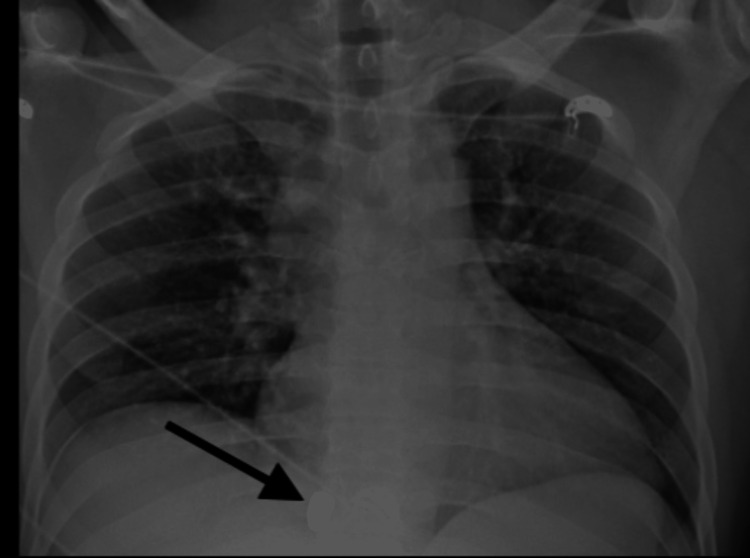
Supine AP chest radiograph demonstrates ballistic fragment projecting over the right heart. AP: anterior posterior.
